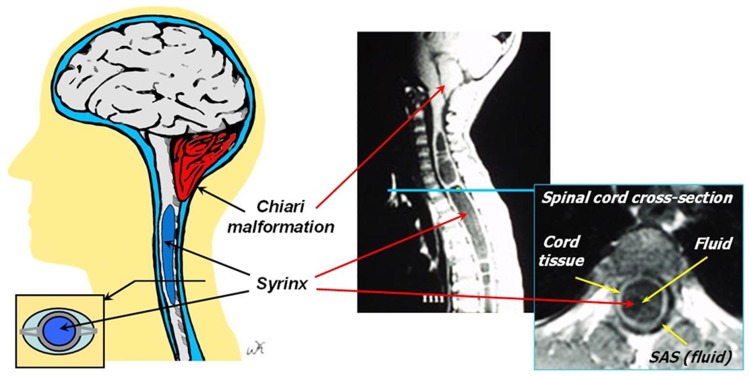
Simplistic representation and corresponding MR images (T1) of the CSF system around the brain and cervical spine for a Chiari patient with syringomyelia showing location of the syrinx (blue region in center of spinal cord) and tonsillar herniation (red at base of brain).
